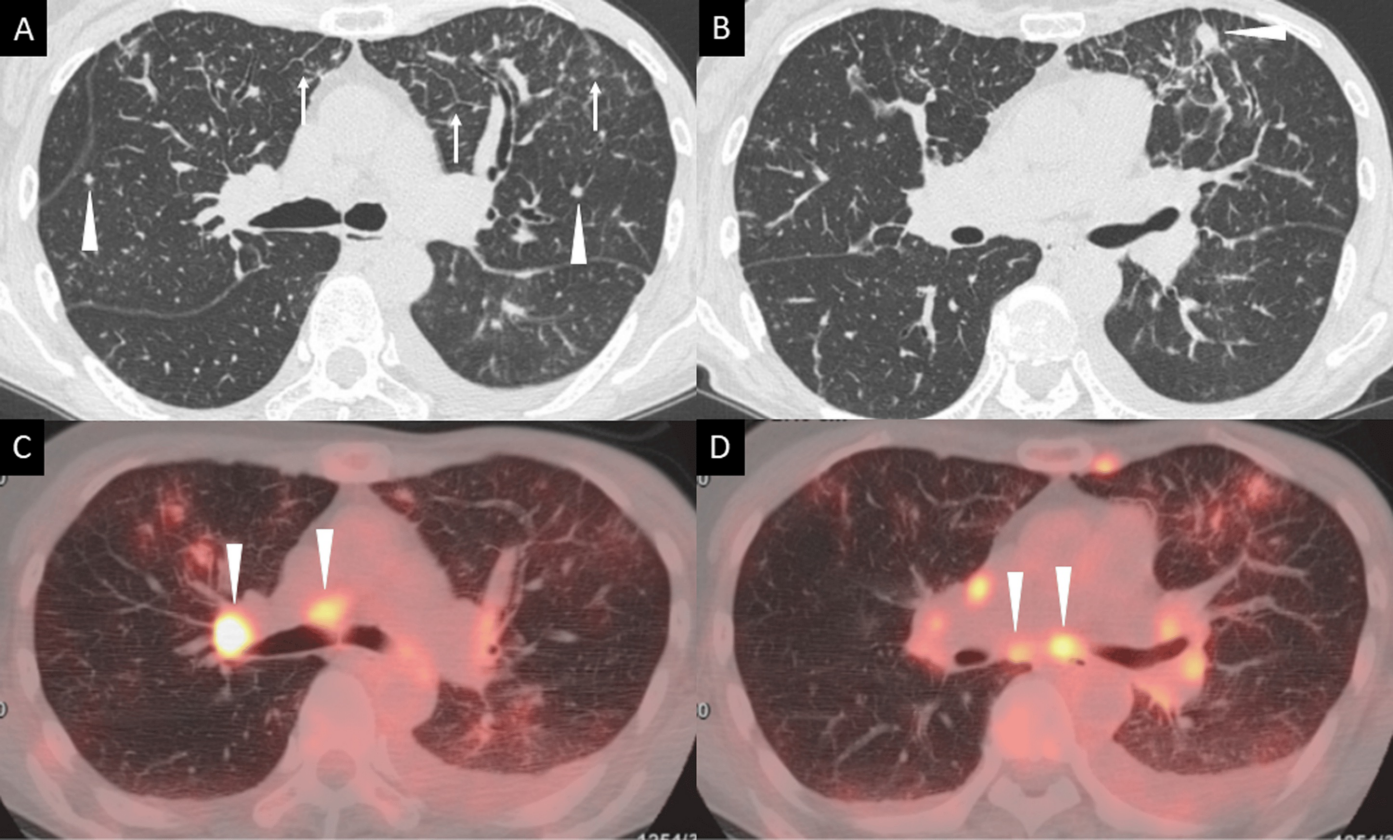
Chest CT and PET/CT findings of case 2 (A) Chest CT image showing multiple pulmonary nodules measuring 3–9 mm in diameter (arrowheads) and thickening of the interlobular septa with fine granular opacities (arrows), findings suggestive of carcinomatous lymphangitis. (B) CT image demonstrating a representative pulmonary nodule (arrowhead) corresponding to the largest lesion (9 mm) in the right lung, with additional smaller nodules present bilaterally. (C, D) PET/CT images showing enlargement and abnormal FDG uptake in multiple LNs, including stations 4R and 7 (arrowheads). Strong FDG accumulation is observed in the LNs, whereas the pulmonary lesions show only mild uptake. CT, computed tomography; PET/CT, positron emission tomography/computed tomography; FDG, fluorodeoxyglucose
